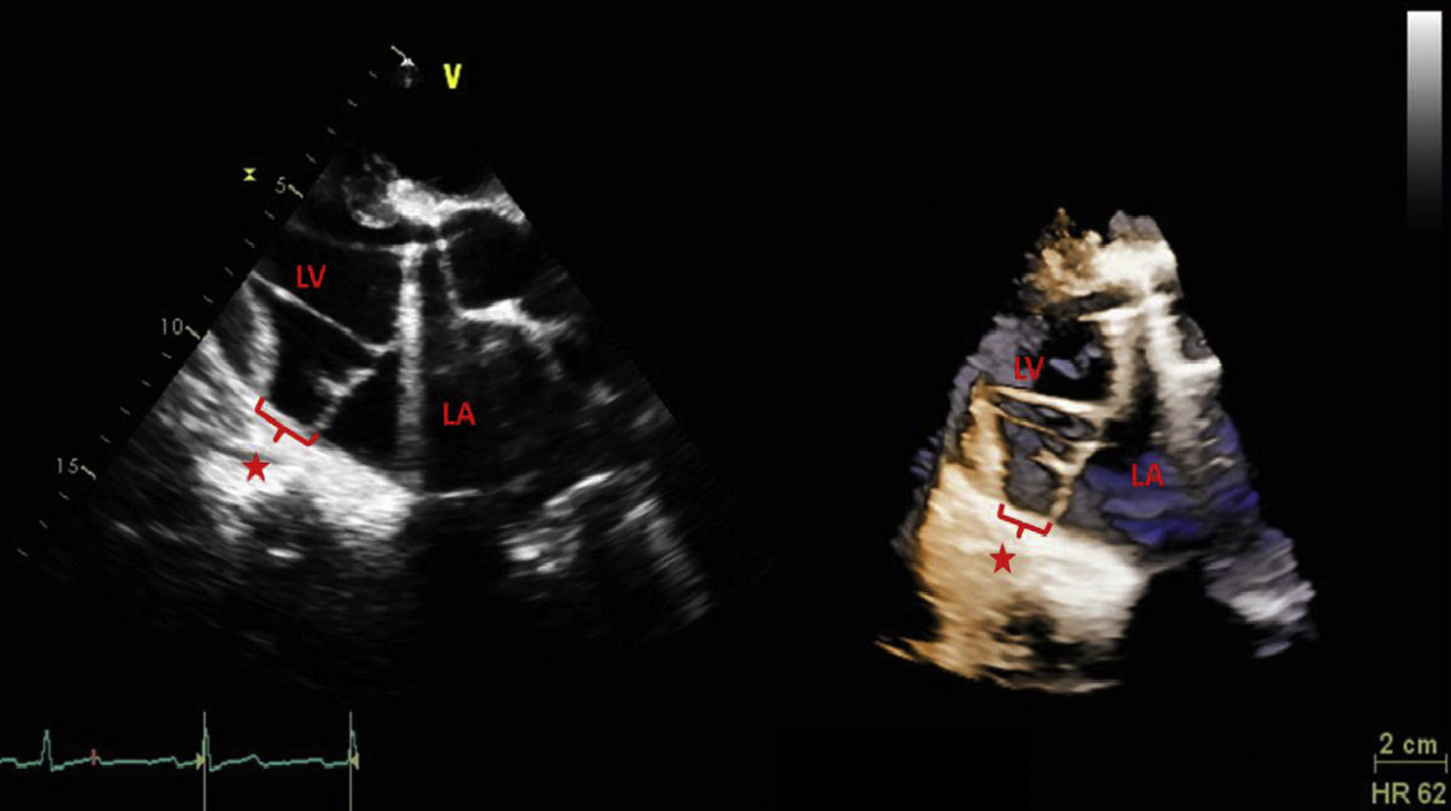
Parasternal long-axis view demonstrating MAD (*red star*) in both two-dimensional (A) and three-dimensional (B) echocardiography. Maximal disjunction distance is indicated by the red bracket. *LA*, Left atrium; *LV*, left ventricle.
